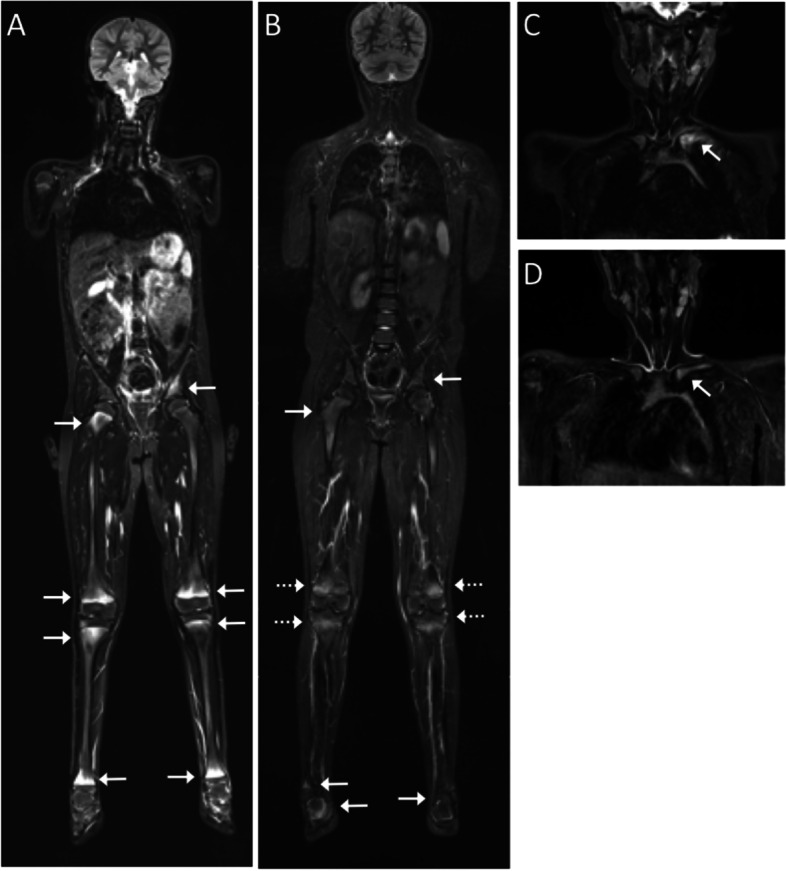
Whole-body MRI following one year of pamidronate treatment. Representative MRI images of a 7-year-old girl diagnosed with chronic non-bacterial osteomyelitis (CNO) and imaging following one year of pamidronate treatment. A Pre-pamidronate whole-body magnetic resonance imaging (WBMRI) short tau inversion recovery (STIR), coronal image shows multiple areas of bone marrow edema as signs of radiologically active lesions (RAL) (white thin arrows). B Post-pamidronate coronal WBMRI STIR image showed resolution of RAL in the left acetabulum and regression of RAL in the right femur. Assessment of RAL in the distal femur and proximal tibia is challenged by bisphosphonate bands (dotted arrows) C Pre-pamidronate and D post-pamidronate coronal MRI STIR images of the clavicles showing post-treatment regression of bone marrow edema and surrounding soft tissue edema
